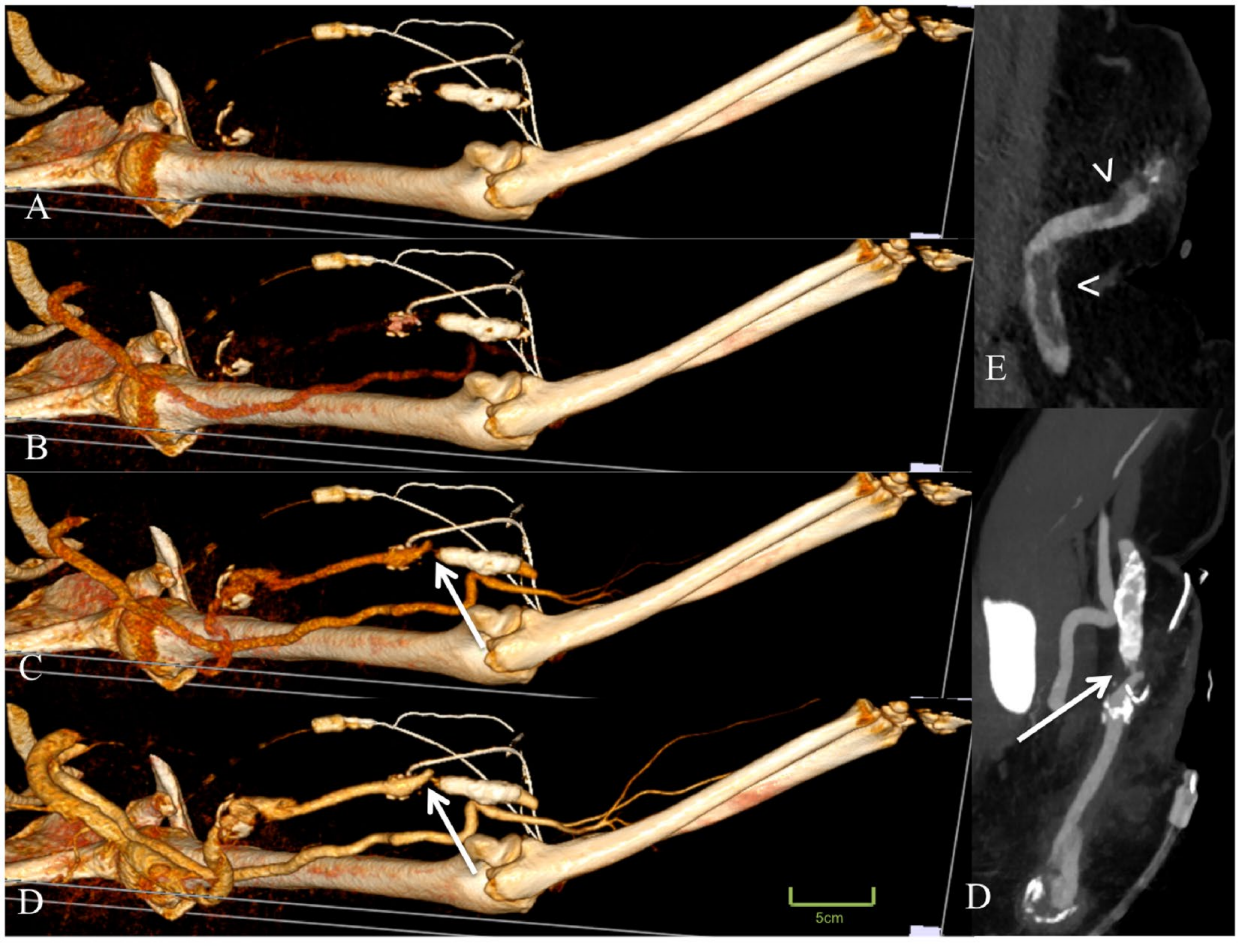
Displayed is a 50-year old female patient with a complex arterio-venous graft and status post multiple revisions, with a new dysfunction of her arterio-venous graft. Time-resolved dynamic CT angiography displayed a high-grade stenosis of the efferent vessel (arrows in C and D). In addition, a thrombus could be detected in the subclavian vein (arrow heads in E).
